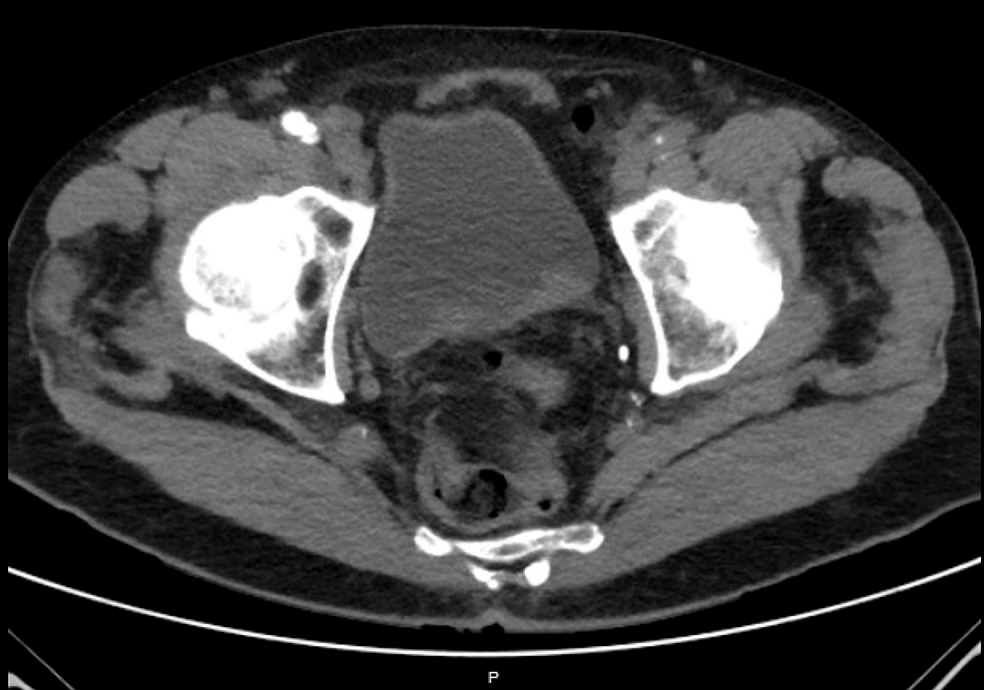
CT urogram showing bladder lobulated polypoid lesion
